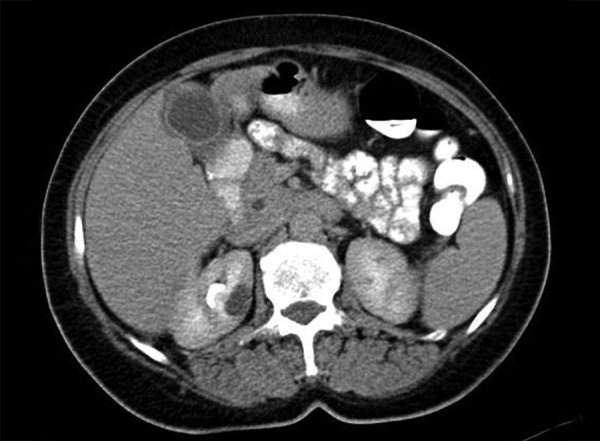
Magnetic resonance imaging of abdomen
